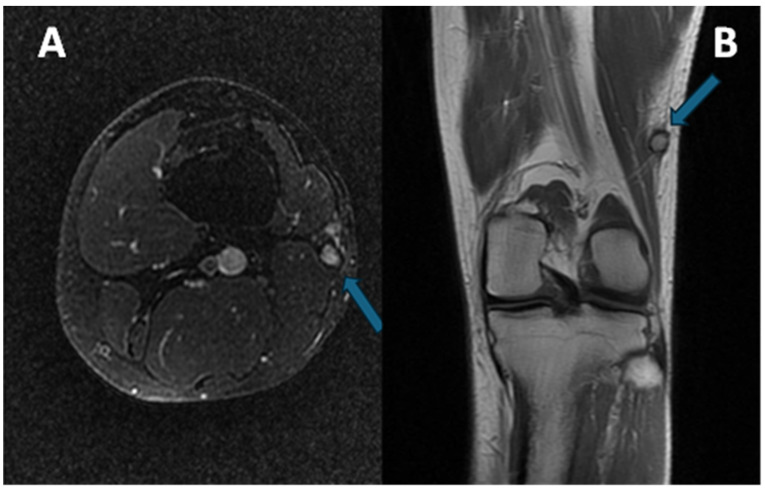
(A) Axial at-saturated T2-weighted imaging showing a small CCS of the left thigh (arrow): note the smooth margins, minimal perilesional edema, and deep location close to the vastus lateralis tendon. (B) Coronal T1-weighted imaging notes the slight hyperintensity of the T1w signal (arrow) compared to the normal muscle. A pseudocapsule, with low signal intensity, is detectable in both sequences.
